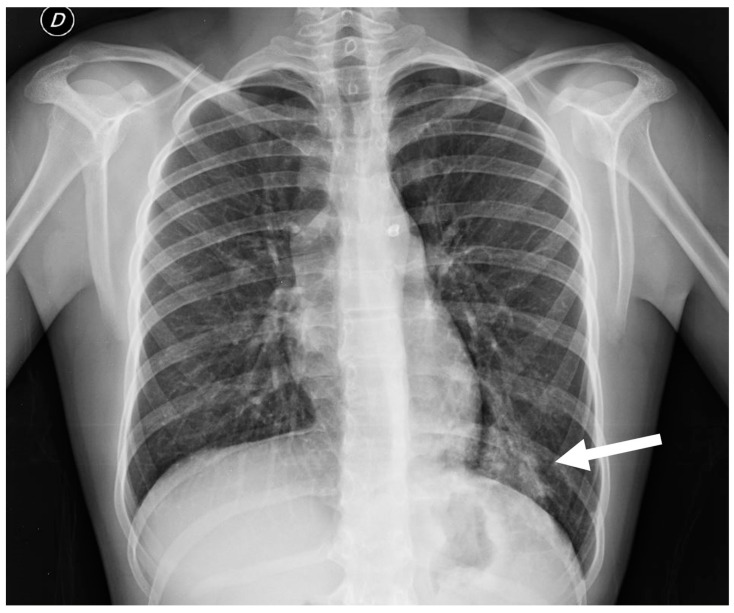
Chest radiograph of a 17 year old boy shows focal lung masses within the left lower lobe with microcystic appearance due to recurrent infection, suggesting an intra-lobar sequestration (arrow).
